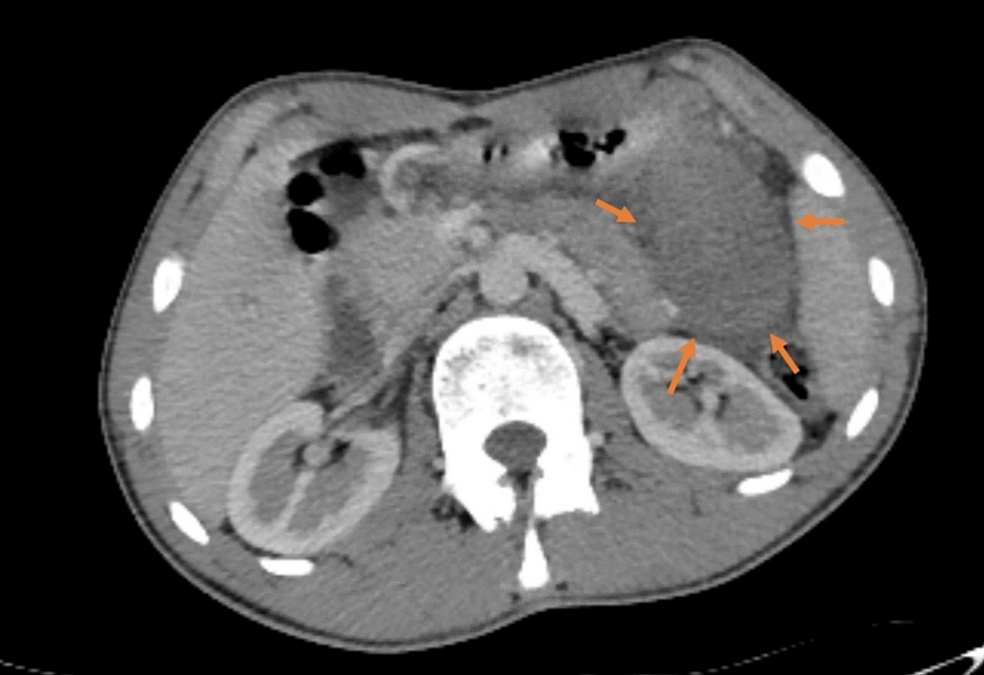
Axial contrast-enhanced CT image shows a well-defined hypodense mass compared to the adjacent spleen and pancreatic tail.
